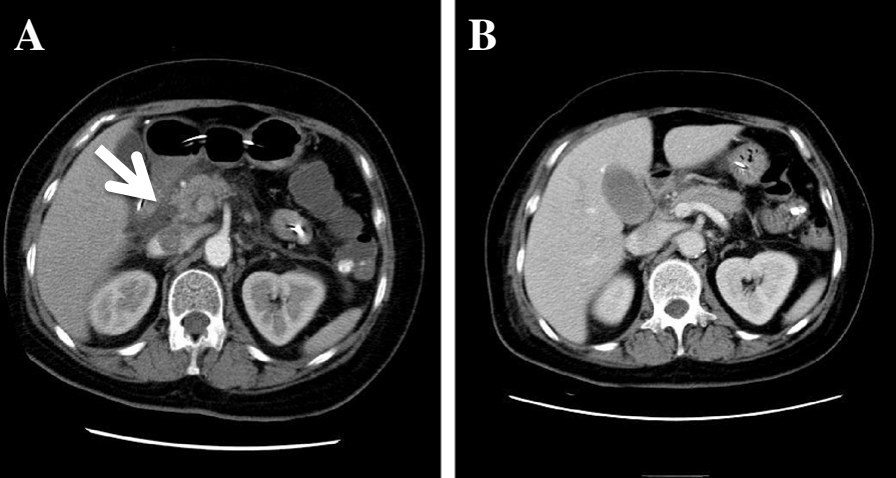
Enhanced abdominal CT scan findings. (A) Enhanced abdominal CT on admission. Around the head of the pancreas, the density of the adipose tissue was increased. There was a fluid collection but no swelling of the pancreas (arrow). (B) Abdominal CT on hospital day 11. The fluid collection has disappeared and there were no cystic changes in the pancreas.
